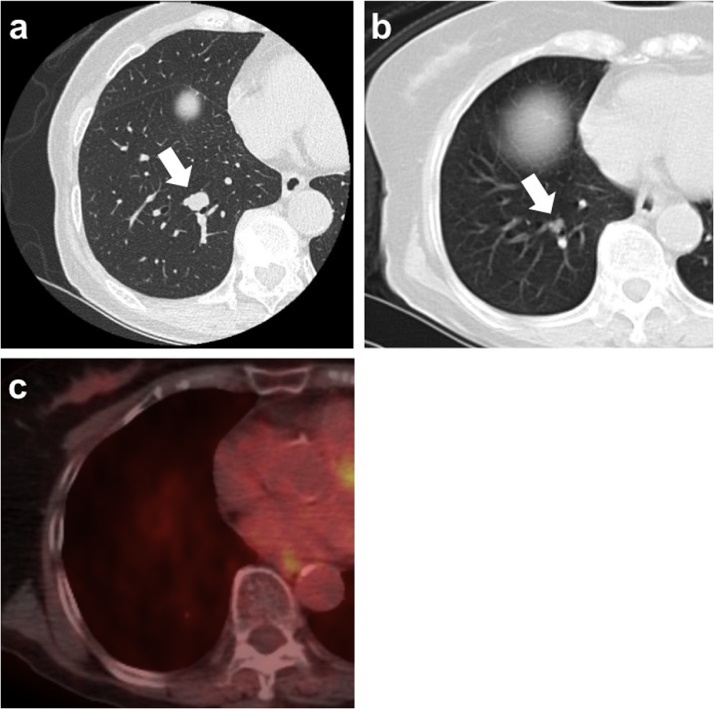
(a) Computed tomography of the chest showing a lung nodule measuring 12 × 10 mm in the right basal segment (arrow). (b) Computed tomography one year prior showing a lung nodule measuring 7 × 6 mm (arrow). (c) Positron emission tomography showing no uptake of 18-fluorodeoxyglucose into the lung nodule.
